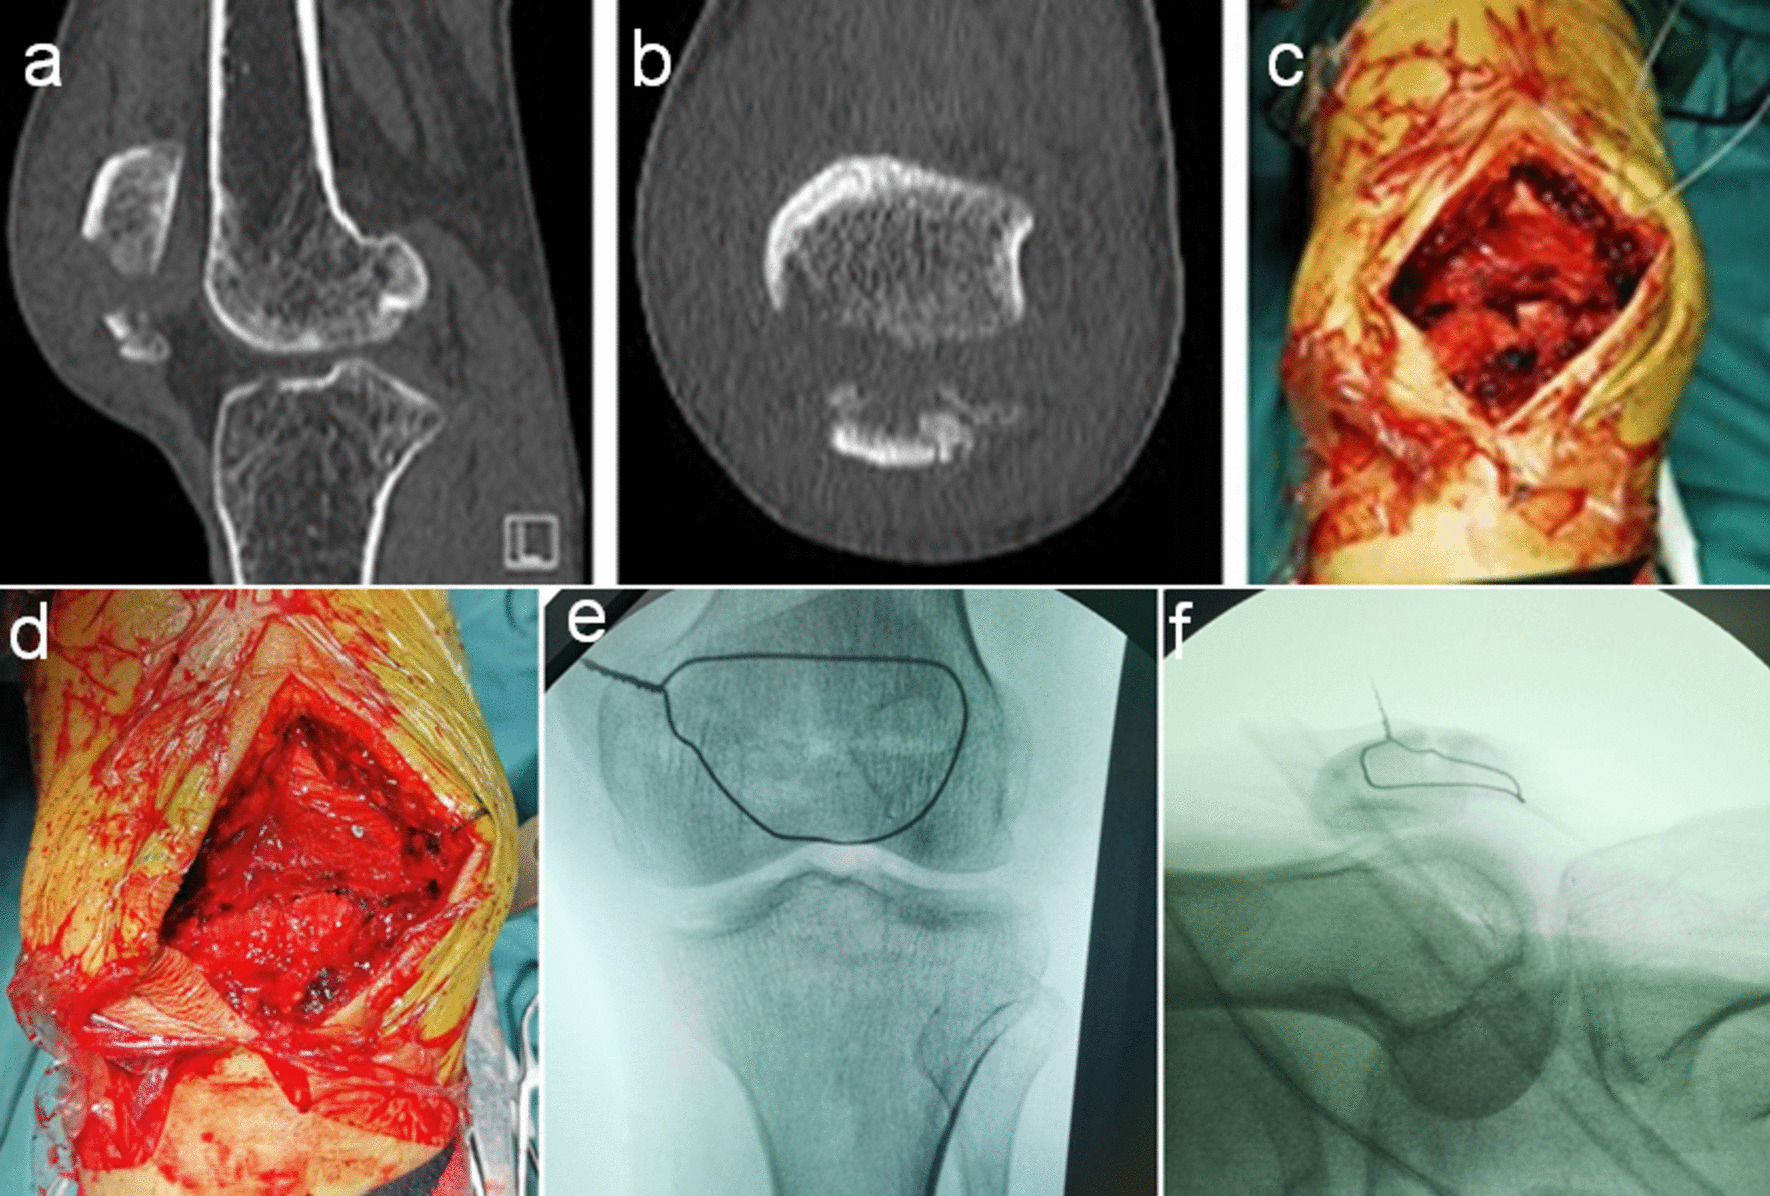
Intra-operative reduction a–b Pre-operative CT examination. c Intra-operative images captured before reduction. d Intra-operative images captured after reduction e–f Intra-operative C-arm fluoroscopy after reduction
